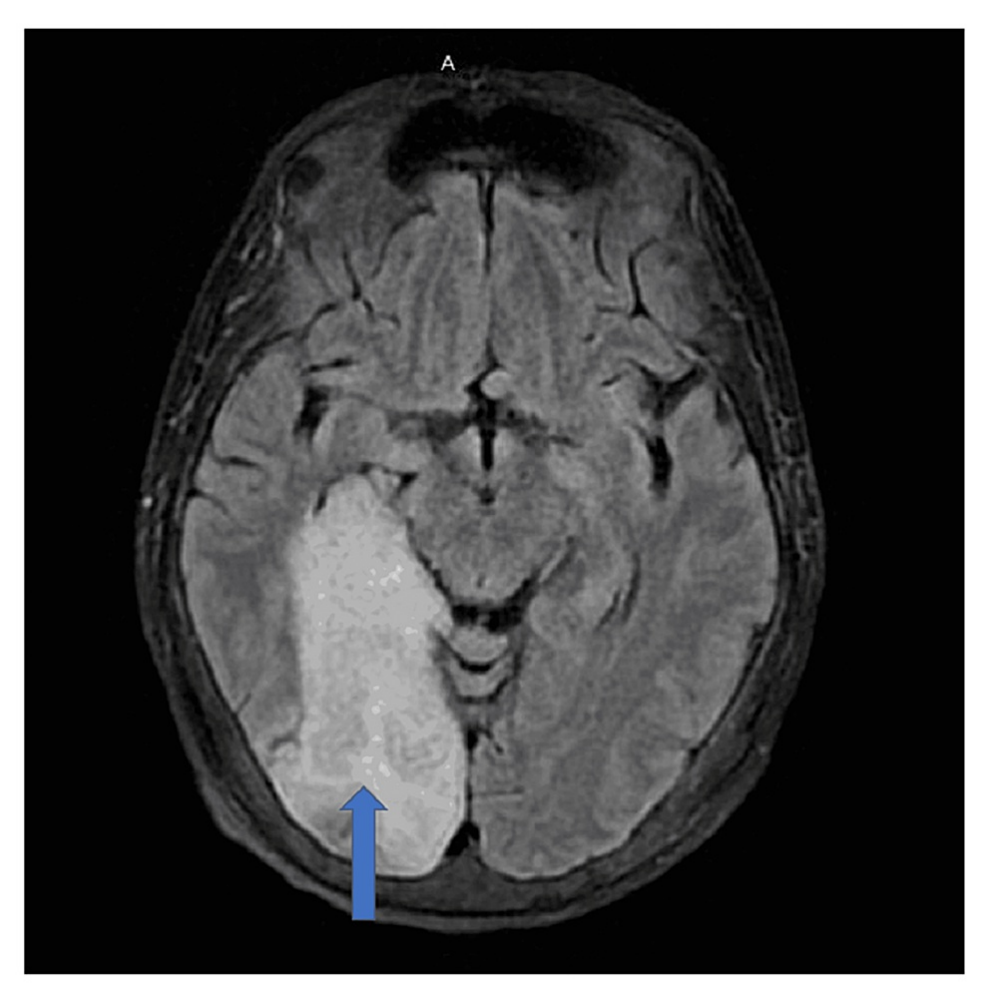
MRI brain showing posterior cerebral artery infarct (blue arrow)
